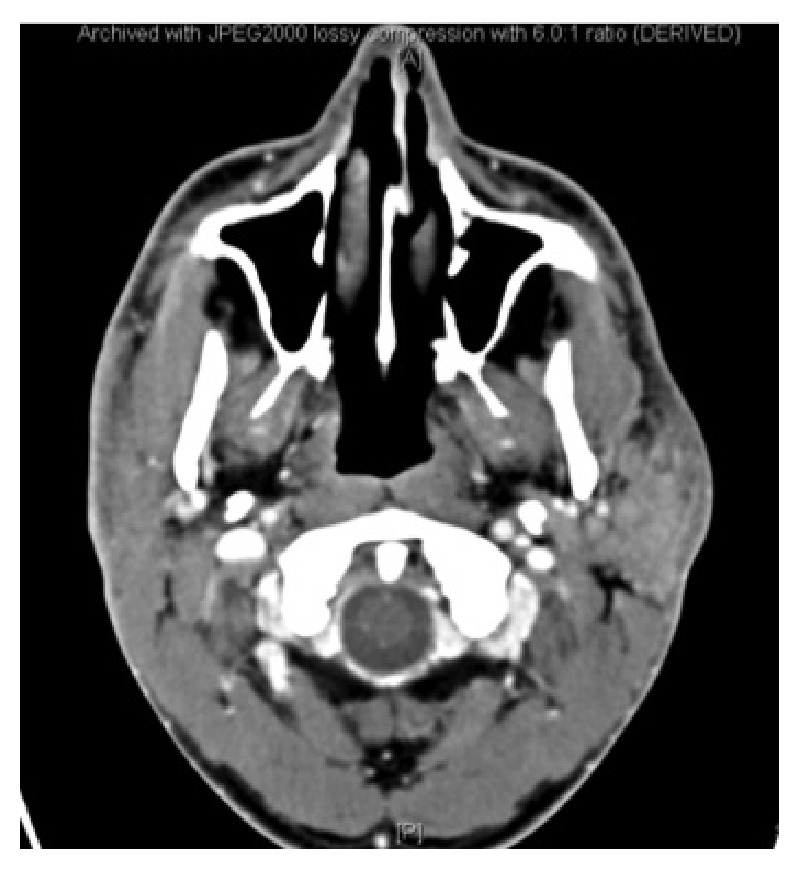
CT axial cut: left parotid gland enlargement with heterogeneous density, multiple different sized intraglandular lymph nodes.
